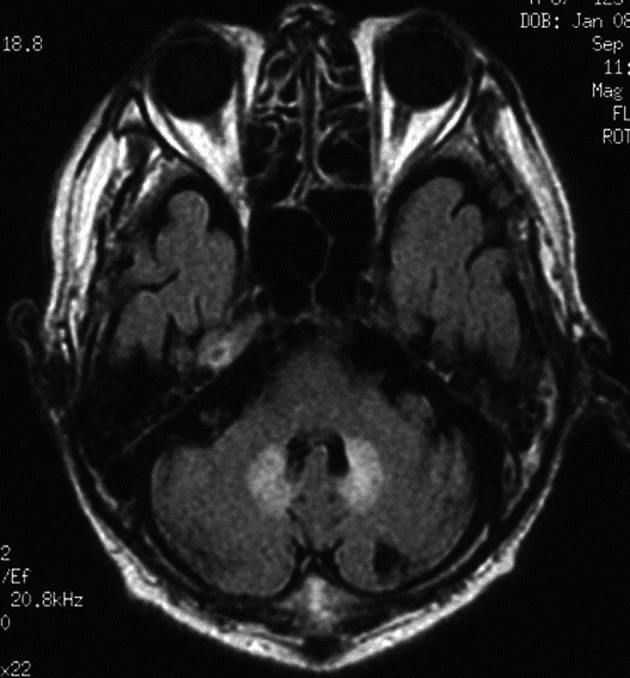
Brain MR imaging (FLAIR) showed hyperintense area of the bilateral basal dendric nuclei which was specific for those of metronidazole-associated encephalopathy.
